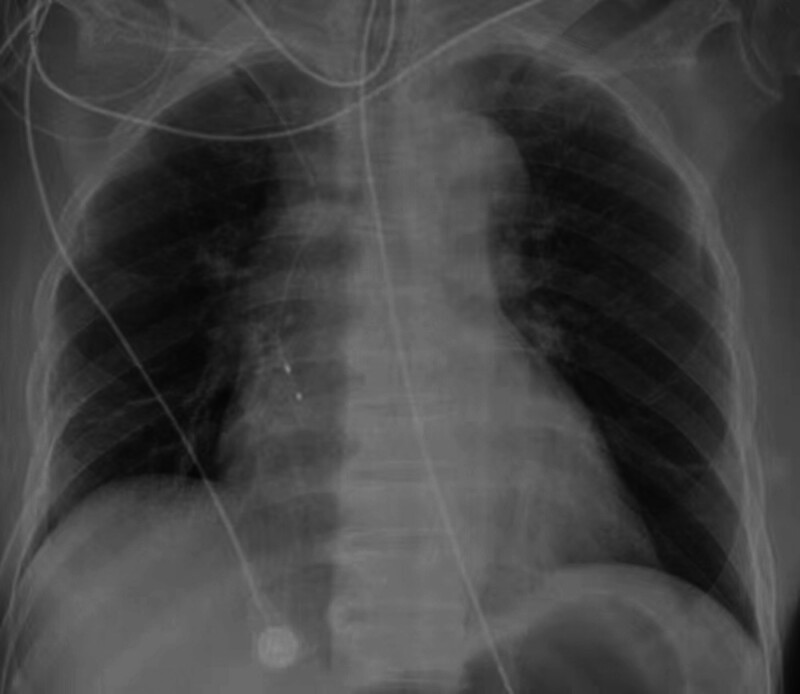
Chest radiograph showing the pacing catheter placed in the right atrium through the subclavian access.
